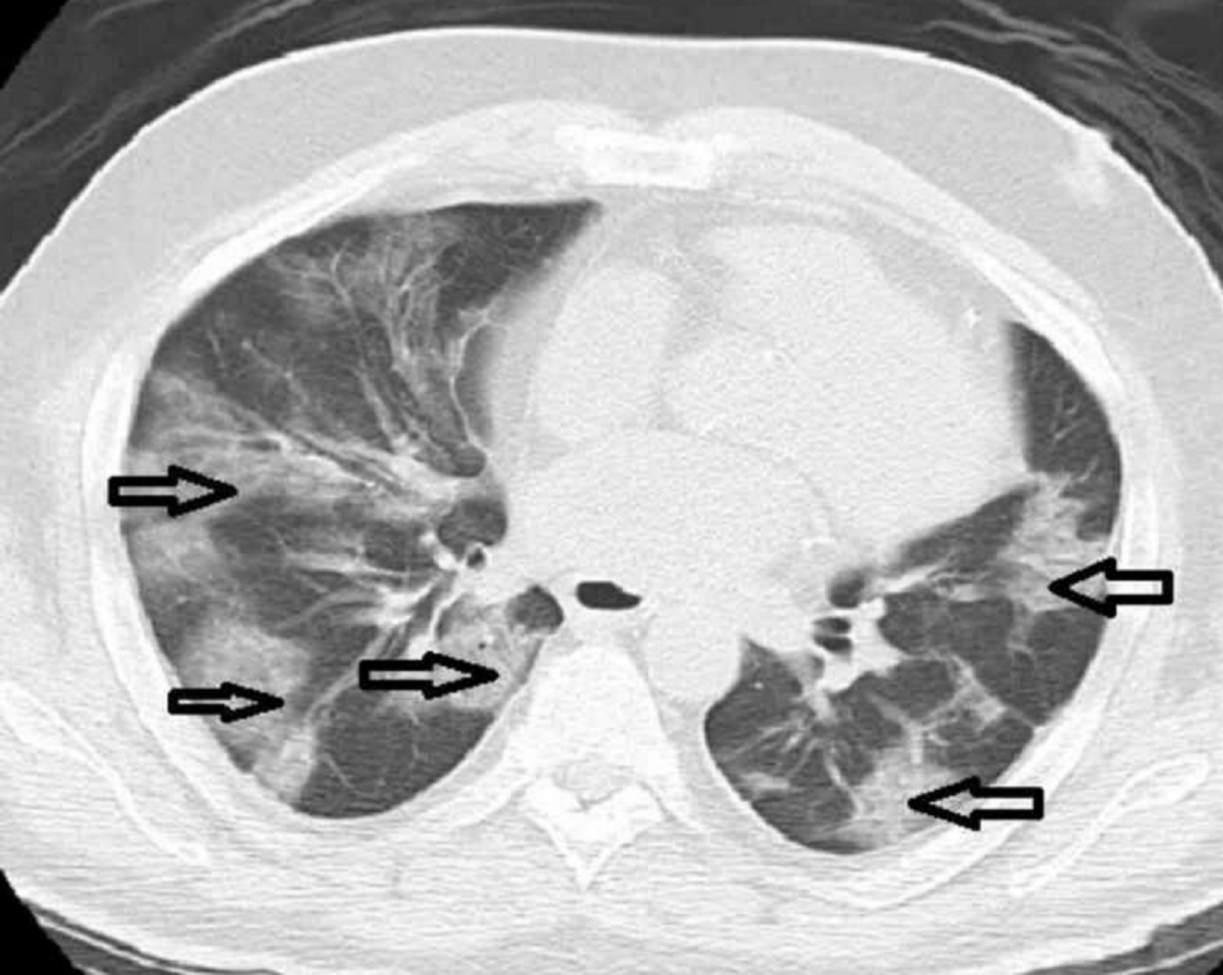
Computed tomography (CT) of the chest without contrast revealed prominent multifocal pneumonia and multiple ground-glass airspace opacities throughout all lung fields
